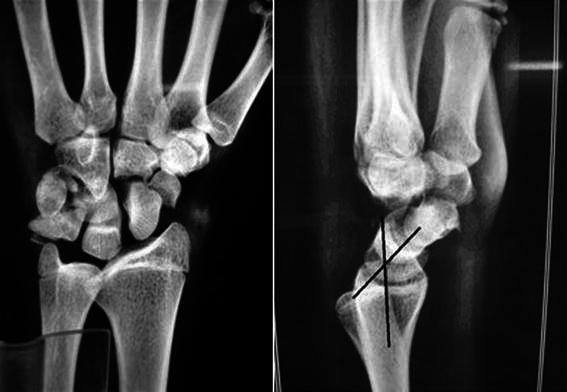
Preoperative radiograph showing fracture of the scaphoid, capitate, and triquetrum, with perilunate dislocation. The radiolunate angle is marked in the figure
